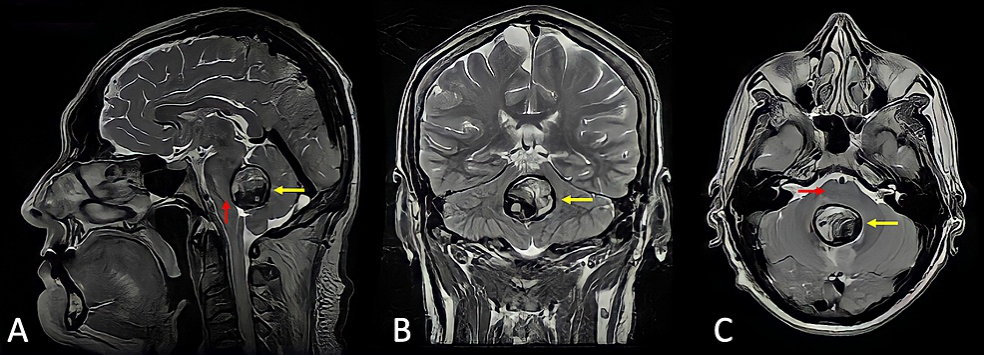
Magnetic resonance imaging (A) Sagittal, (B) coronal, and (C) axial T2 weighted sequence, which shows a 27-mm solid, heterogeneous, and occipital lesion fully occupying the fourth ventricle (yellow arrows). Brainstem displacement and edema can be observed (red arrows).
